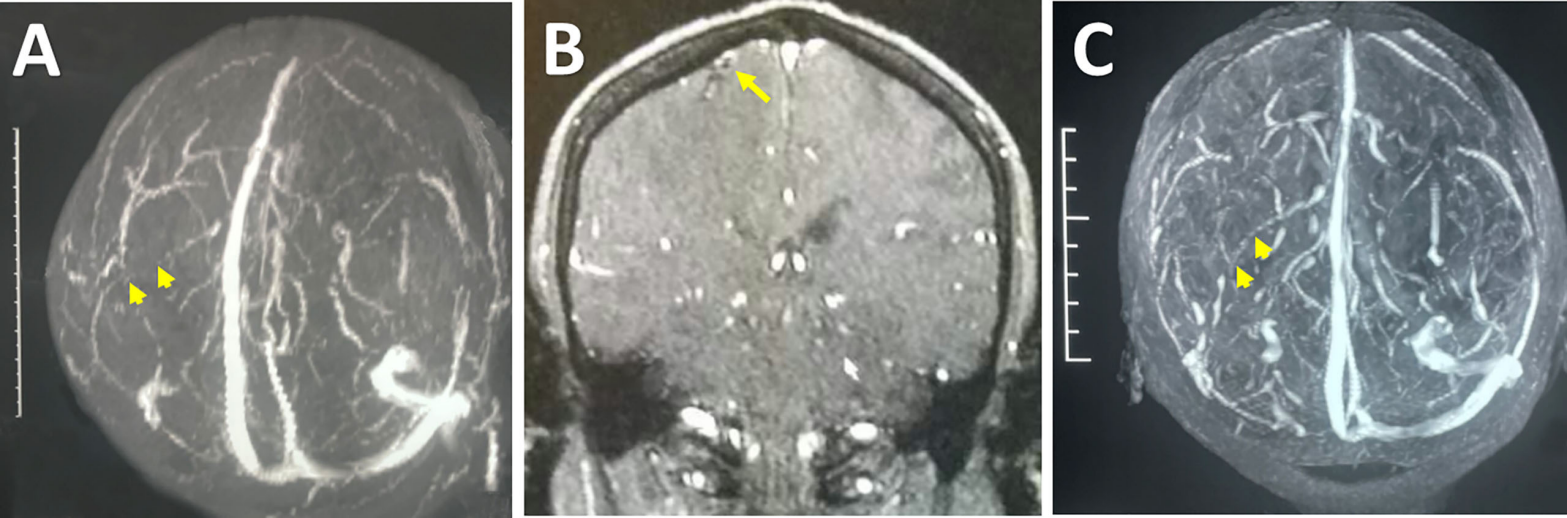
Radiological images indicating the ICVT. Preoperative MRV revealed an absence of flow (arrowheads) in the left central sulcus vein (A). Preoperative contrast MRI showed an intraluminal filling defect at the site where the deep branch of the right central sulcus vein enters the main trunk (arrow), likely indicative of ICVT (B). Follow-up MRV showed the recanalization (arrowheads) of the right central sulcus vein after surgical thrombectomy (C).
